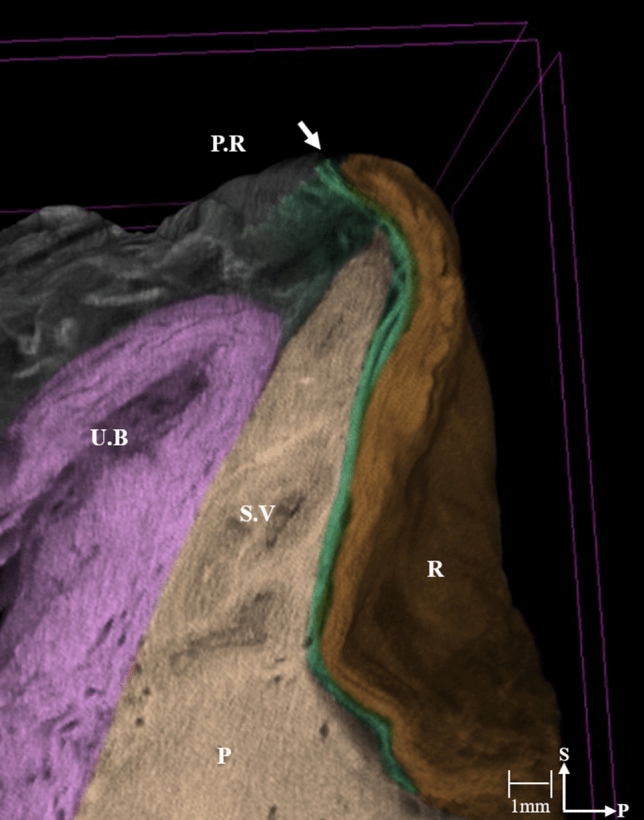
Three-dimensional anatomy of the hemi-pelvis reconstructed from micro-computed tomographic images. The arrow in in the micro-computed tomographic image indicates the deepest point of the rectovesical pouch where the DVF extends. (DVF; green). The 3D morphology of the DVF is also shown as a movie in Supplemental Video 1. PR, peritoneal reflection; UB, urinary bladder; SV, seminal vesicle; P, prostate; R, rectum; S, sagittal; P, posterior.
